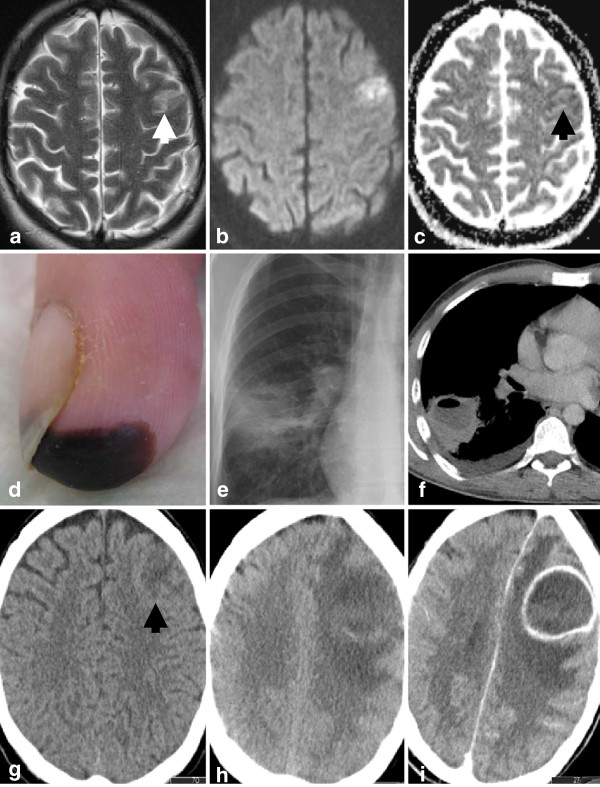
a) T2 weighted axial image displayed a hyperintense cortical signal alteration in two adjacent gyri of the left supplementary motor cortex (white arrow).b) This lesion was hyperintense in the axial diffusion weighted image (b = 1000 sec/mm^2^. Although it showed only minimally lowered ADC values (Apparent Diffusion Coefficient, black arrow, c), these findings were primarily consistent with an acute infarction. d) The index finger of his left hand showed a typical Janeway lesion highly indicative of a septic-embolic focus. Chest X-Ray (e) and computed tomography (f) showed a large fluid and air containing process in the right lower lobe consistent with a septic lung abscess. g) A computed tomography two days later revealed a now well demarcated lesion (native scan in axial orientation, black arrow). h) A further nine days later, after clinical deterioration a repeated scan revealed a large left frontal mass (native scan) with ring-like enhancement after i.v. administration of iodine contrast media (i). The findings were now typical for a brain abscess.
